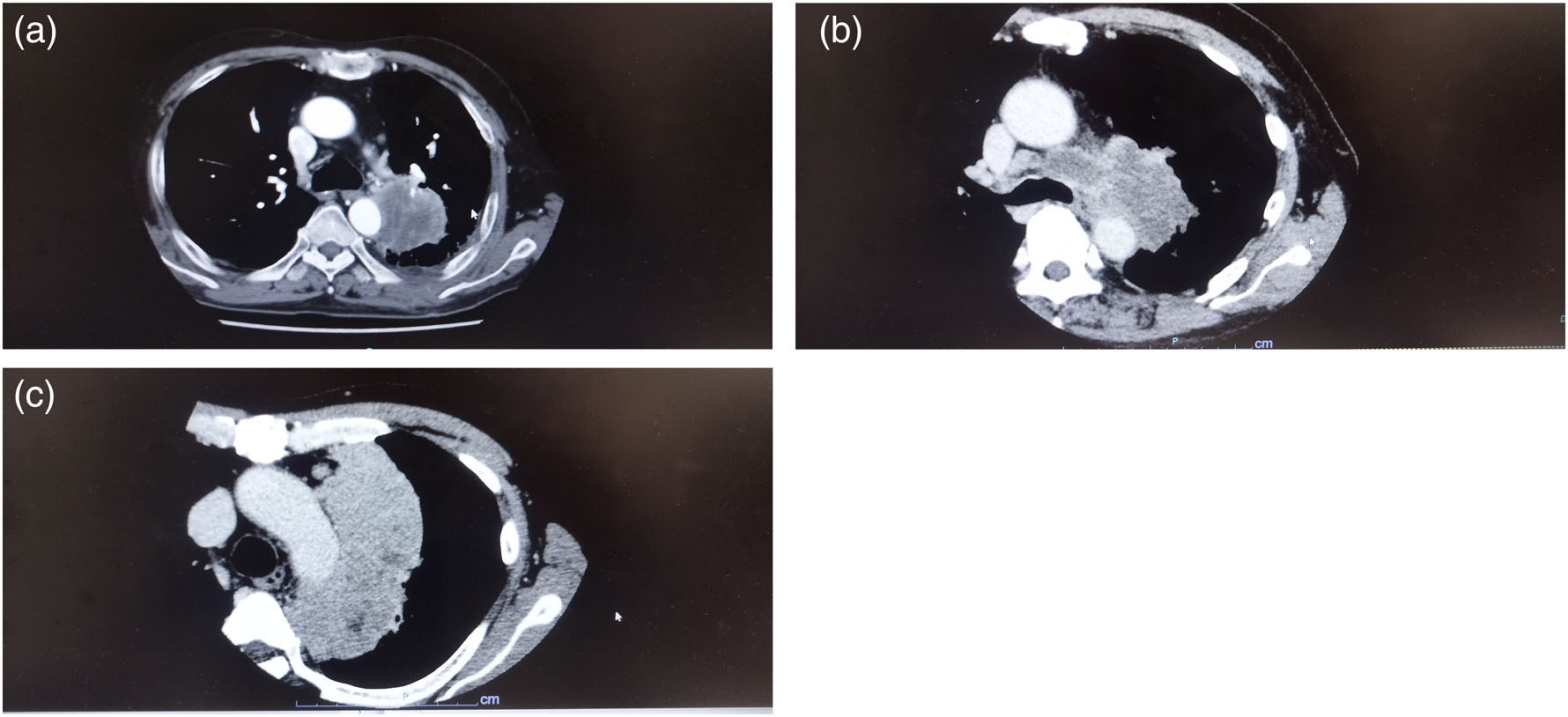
Chest computed tomography (CT) findings due to the tumor. (a) Approximation to the aorta by >90°. (b) Aortic depression. (c) Loss of fatty tissue between tumor and mediastinum.
